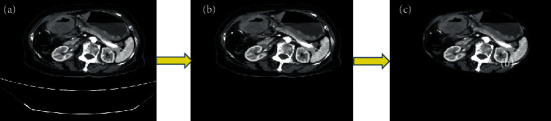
Display of preprocessing results of stomach CT images: (a) original image; (b) image of the examination bed removed; (c) image of the subcutaneous fat removed.
